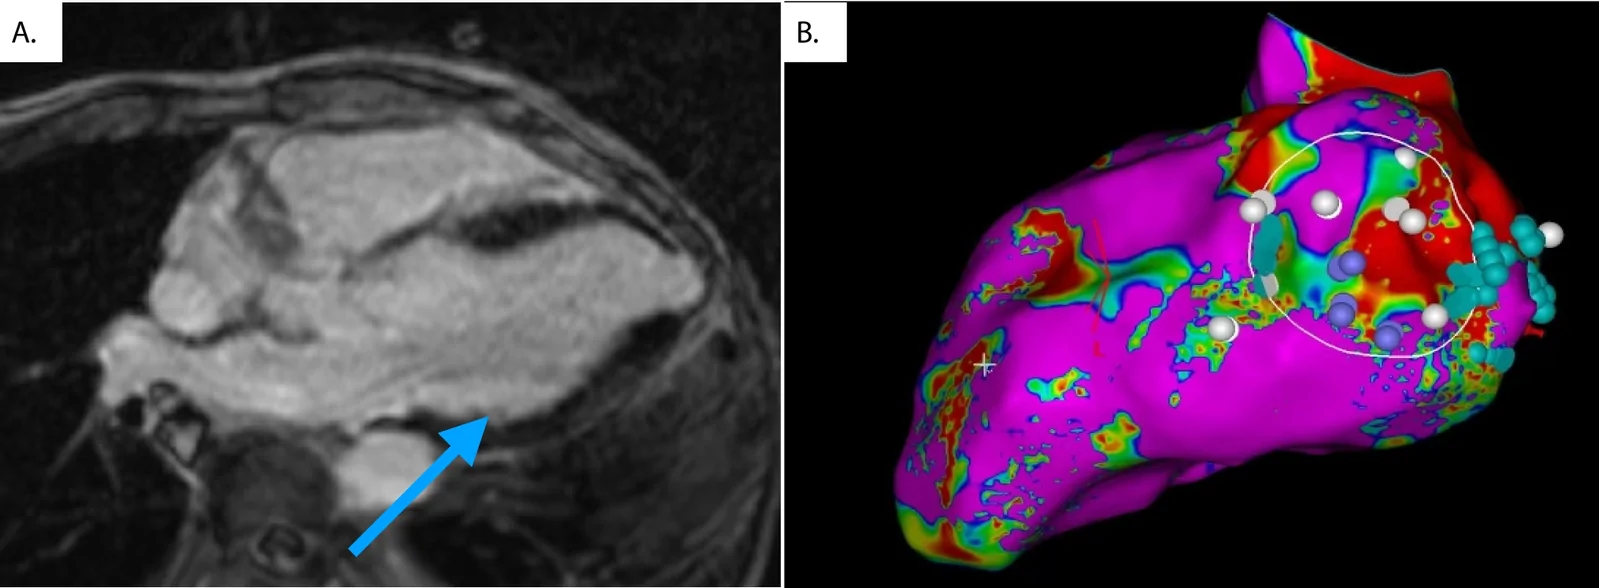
A. MRI showing lateral gadolinium enhancement, which correlated B. Endocardial low voltage and site of successful VT ablation
